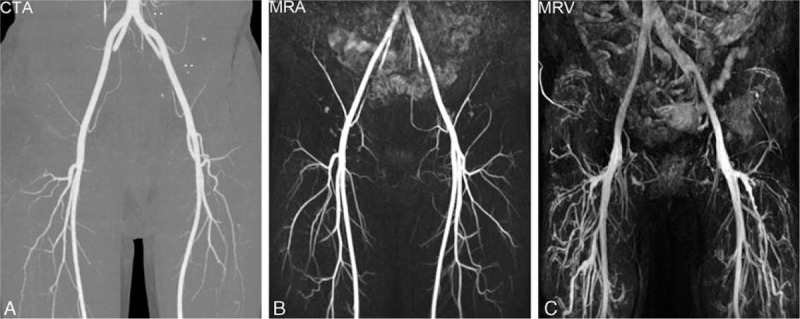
(A) CTA image rated as excellent image quality. (B) Arterial phase TWIST image rated as excellent image quality. The arterial SNR is high with small branches clearly depicted. (C) Venous phase TWIST image with diagnostic image quality and no arterial contamination. CTA = computed tomography angiography, MRA = magnetic resonance angiography, MRV = magnetic resonance venography.
